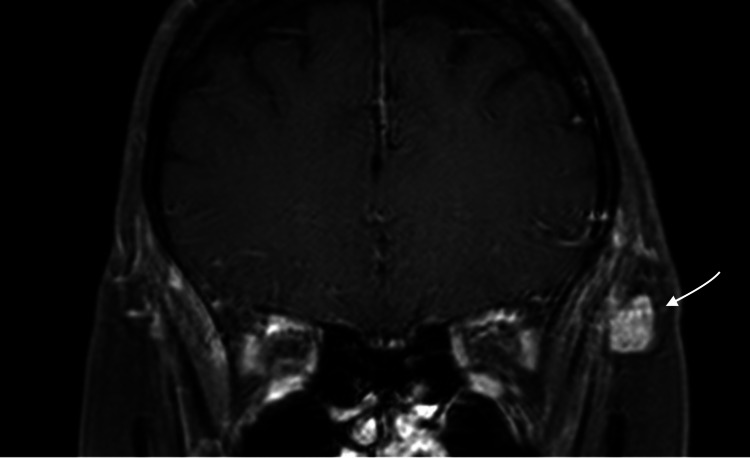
MRI with contrast imaging, coronal view The arrow shows the identified lesion with no calcification
